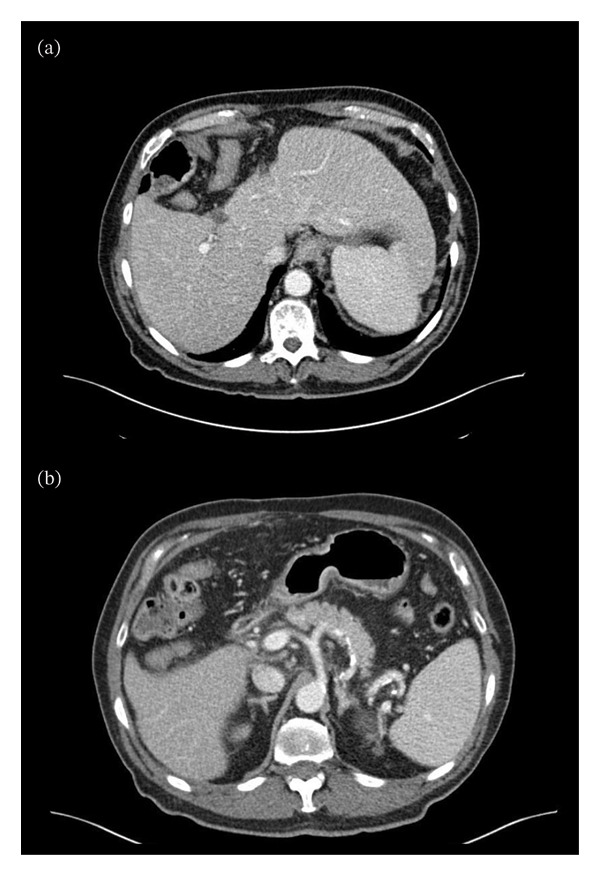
Computed tomography (CT) (image (a)) demonstrates minor hepatomegaly extending to the left upper quadrant. No hepatic lesions are present. Spleen size is at the upper limit of normal to mildly enlarged. Image (b) shows peripancreatic fat stranding and edema consistent with acute pancreatitis.
